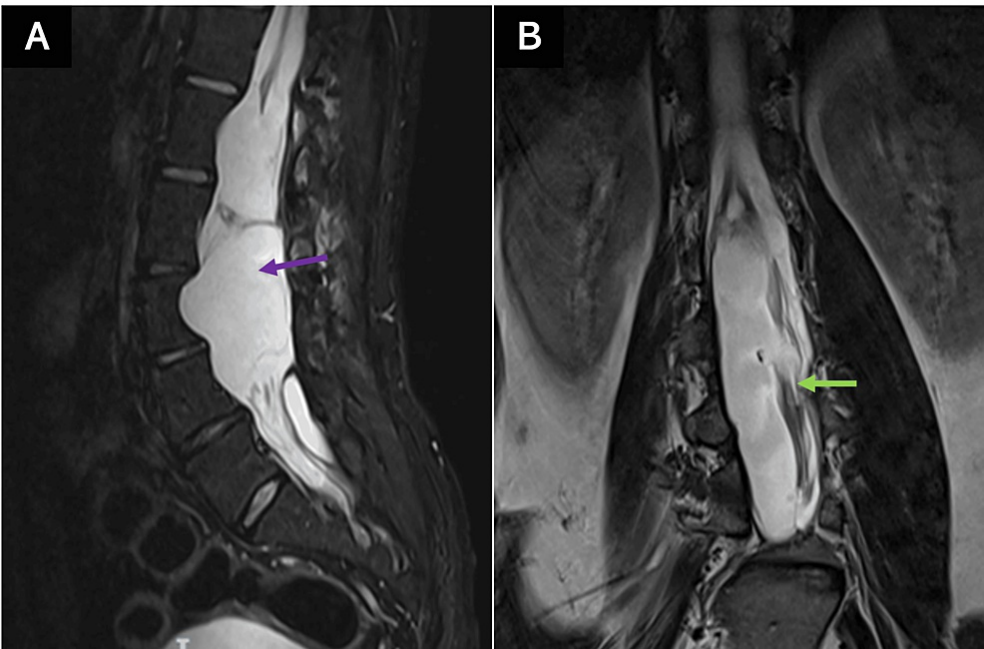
Postoperative MRI (A) Postoperative sagittal T2 MRI without residual tumor, with occupation of cerebrospinal fluid in the site where the tumor was located (purple arrow). (B) Postoperative sagittal T2 MRI cauda equina displacement (green arrow). MRI: magnetic resonance imaging
